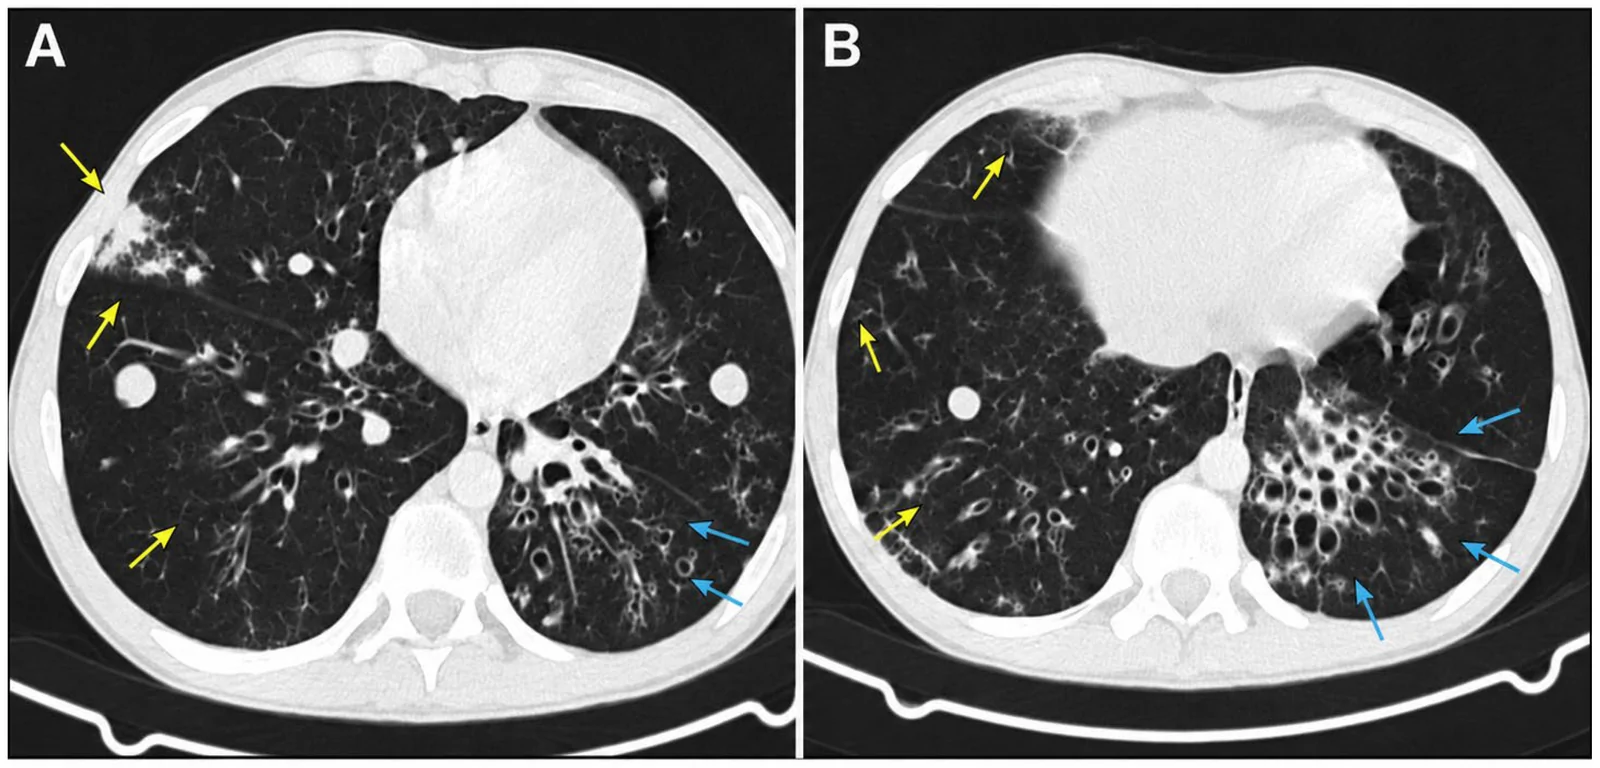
Thoracic CT findings in a patient with DOCK8 deficiency and pulmonary tuberculosis (F8.P2). (A,B) Axial thoracic CT images showing multifocal nodular and patchy parenchymal opacities in both lungs (yellow arrows) and extensive cystic/varicose bronchiectatic changes, predominantly involving the left lower lobe (blue arrows).
